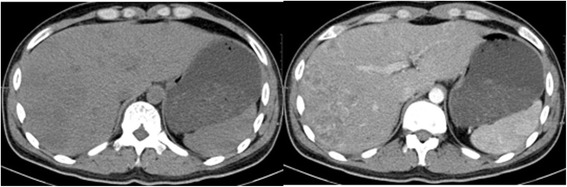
CT showing multiple new masses are present in the liver with peripheral enhancement
